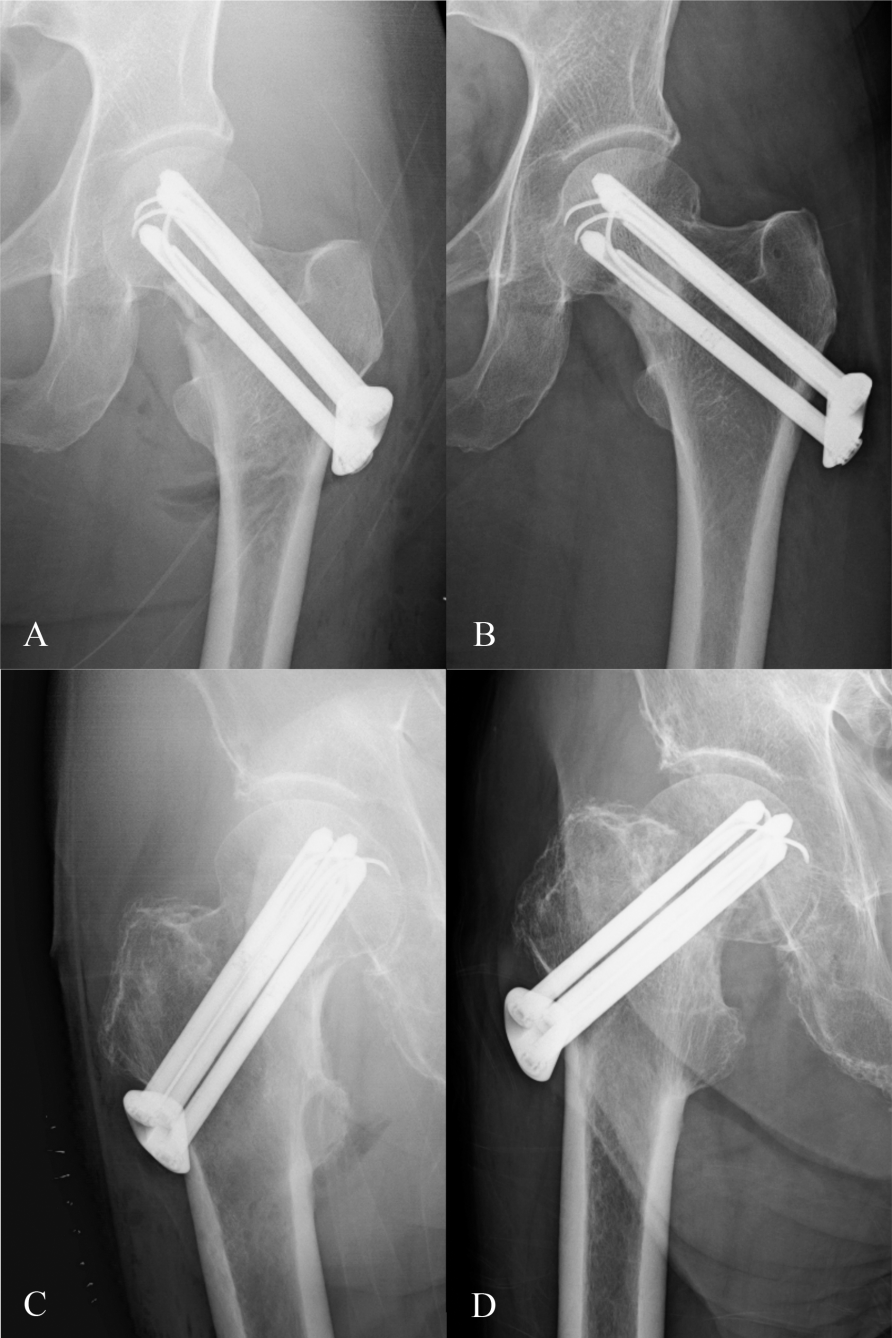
Two typical scenarios of IF. Immediate postoperative X-ray of a 60-year-old woman with an acute displaced femoral neck fracture treated with internal fixation using Pinloc (A) and the corresponding 12-month follow-up X-ray (B), showing fracture healing. Immediate postoperative X-ray of an 80-year-old man with an acute displaced femoral neck fracture treated with internal fixation using Pinloc (C) and the corresponding 6-month follow-up X-ray (D), showing the non-union of femoral neck fracture.
